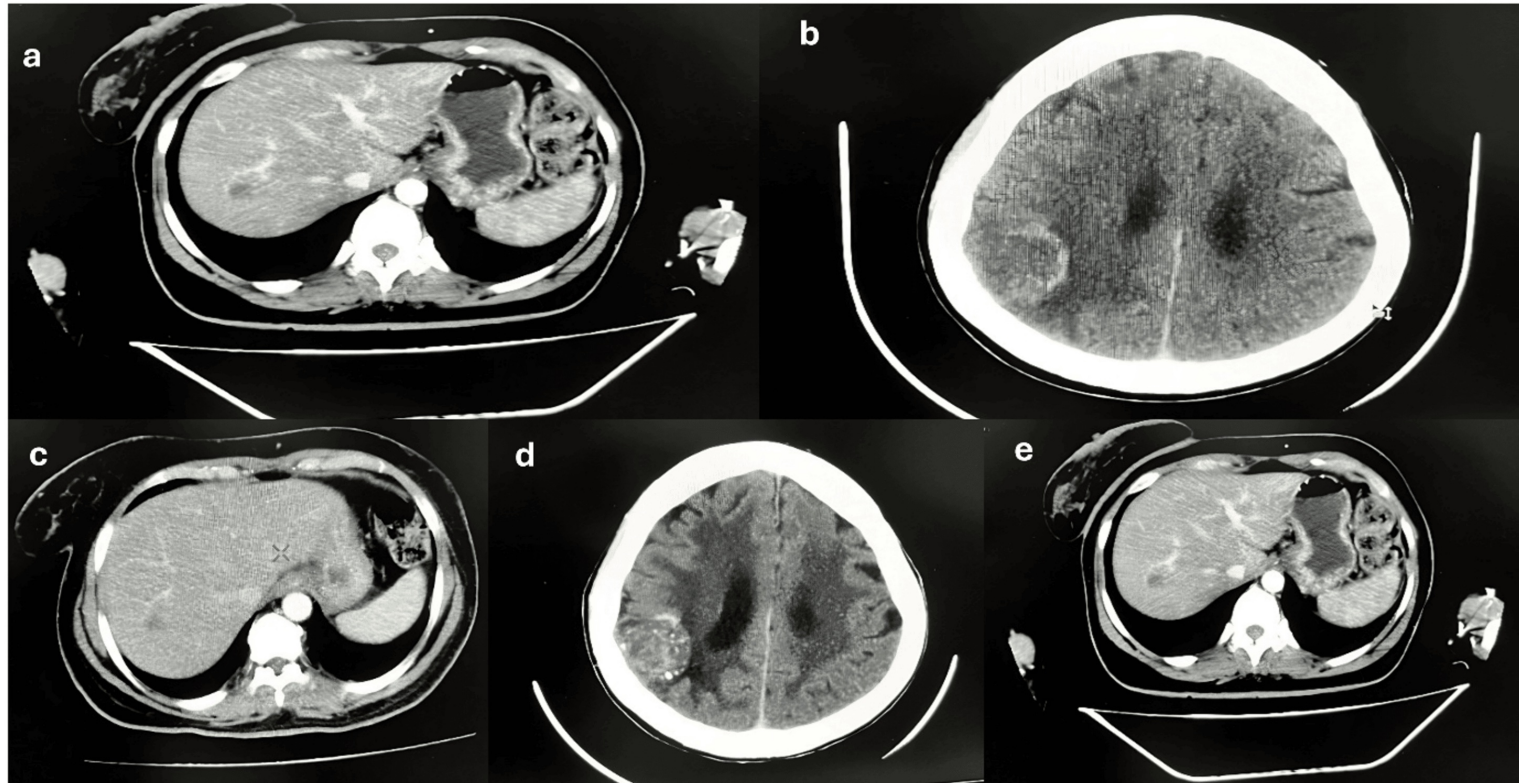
(a) A small enhancing nodule measuring 13 mm in the right lobe in segment VII identified on CECT; (b) a heterogeneously enhancing solid mass lesion measuring 34 x 32 mm in the right post-parietal region with perilesional edema suggestive of a regressed lesion identified via CECT; (c) stable disease observed on CECT; (d and e) both images suggestive of stable disease as observed on CECT. CECT, contrast-enhanced computed tomography.
